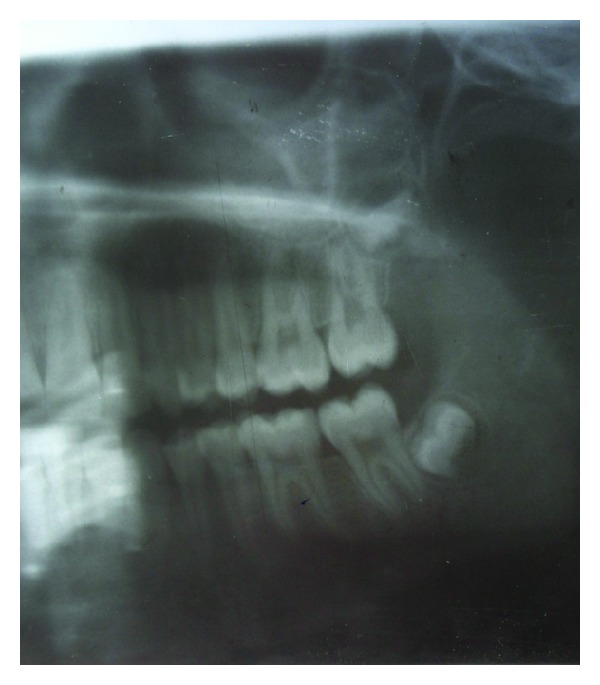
An orthopantomogram showing mottled unilocular radiolucency.
